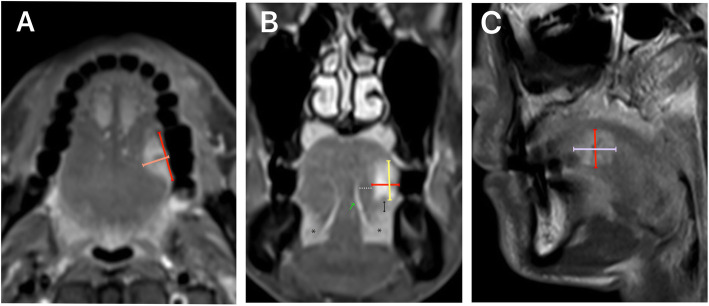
T1W1 MRI showed measurement of MRI-assessed tumor thickness in three dimensions. All reference lines (red line) were defined as the longest tumor diameter in each direction. a. Axial tumor thickness (aTT) (orange line), b. Coronal tumor thickness (cTT) (yellow line). c. Sagittal tumor thickness (sTT) (purple line). In the coronal view, a thin high signal intensity (green arrow line) was used to identify the deep lingual artery and inferior lateral area of high signal intensity (asterisk) was indicated the sublingual space. Paralingual distance was defined as the distance between the lateral aspect next to the deep lingual artery and medial border of the tumor (dotted line). Sublingual distance was determined by measuring the vertical distance between the inferior border of tumor and the sublingual space (black arrow line)
